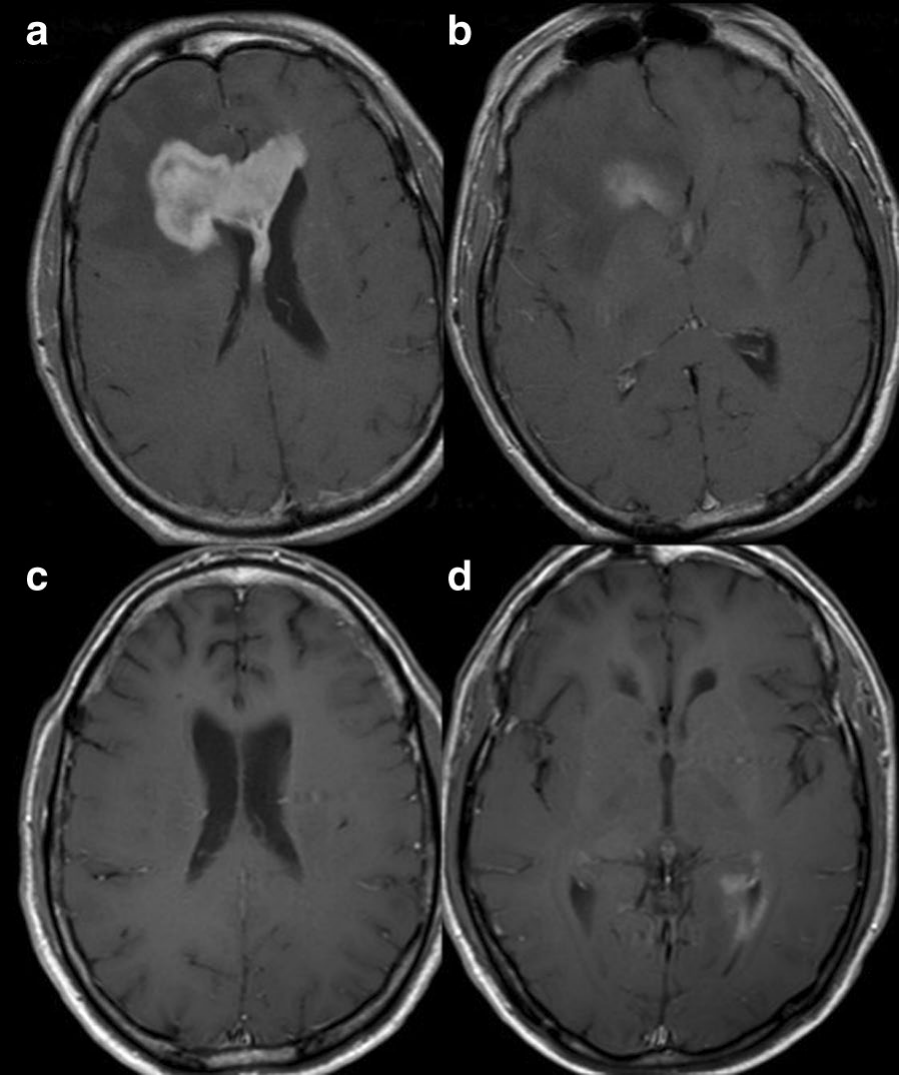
Case 2; Axial T1-weighted post contrast imaging at initial presentation (a, b) and relapse (c, d). Initial presentation shows a localized large enhancing lesion centered in the genus of the corpus callosum (CC) with extension mainly into the right frontal lobe (a, b). After 10 months of HD-MTX treatment there is relapse in the ependymal surface of the left lateral ventricle (d) with complete response in the CC lesion (c)
